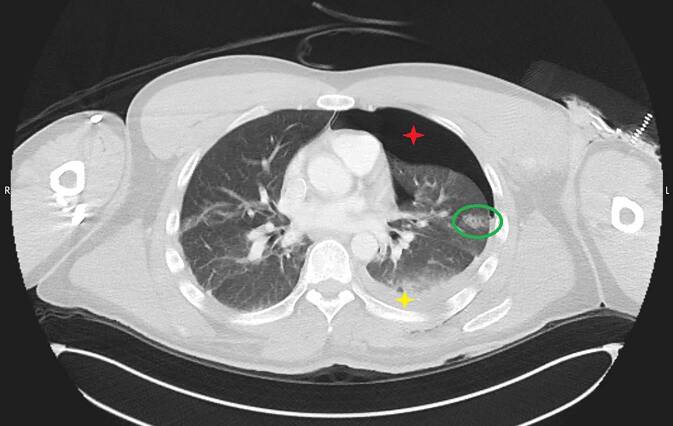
CT des Thorax, axial im Lungenfenster. Lungengewebsverletzung (*Kreis*) und ipsilateraler Hämatopneumothorax (*gelber* bzw. *roter Stern*) nach Auseinandersetzung mit einer Glasscherbe und Verletzung links thorakal (achselhöhlennah)
